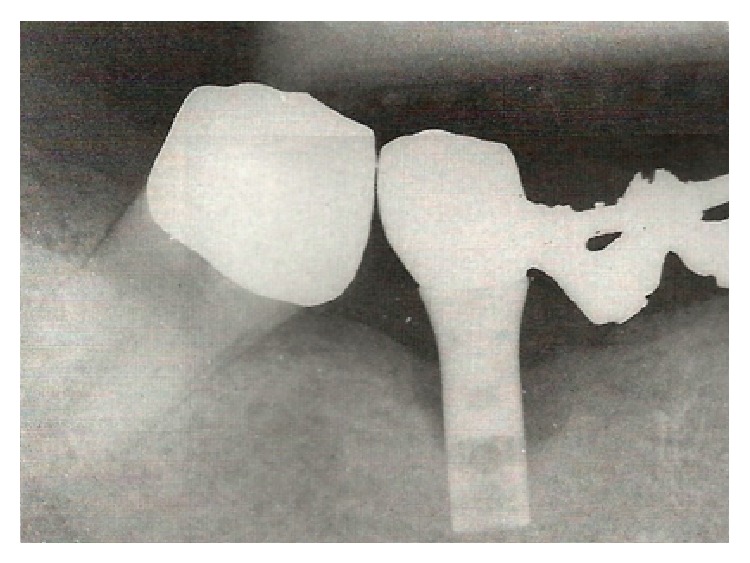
Preoperative intraoral radiography. A typical peri-implantitis crater-like defect was evident.
